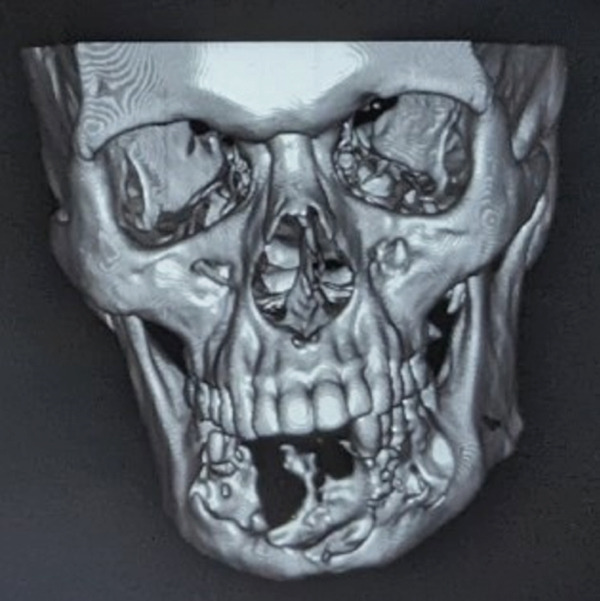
CBCT shows a unilocular well‐defined expansile lesion with a multilocular region.
